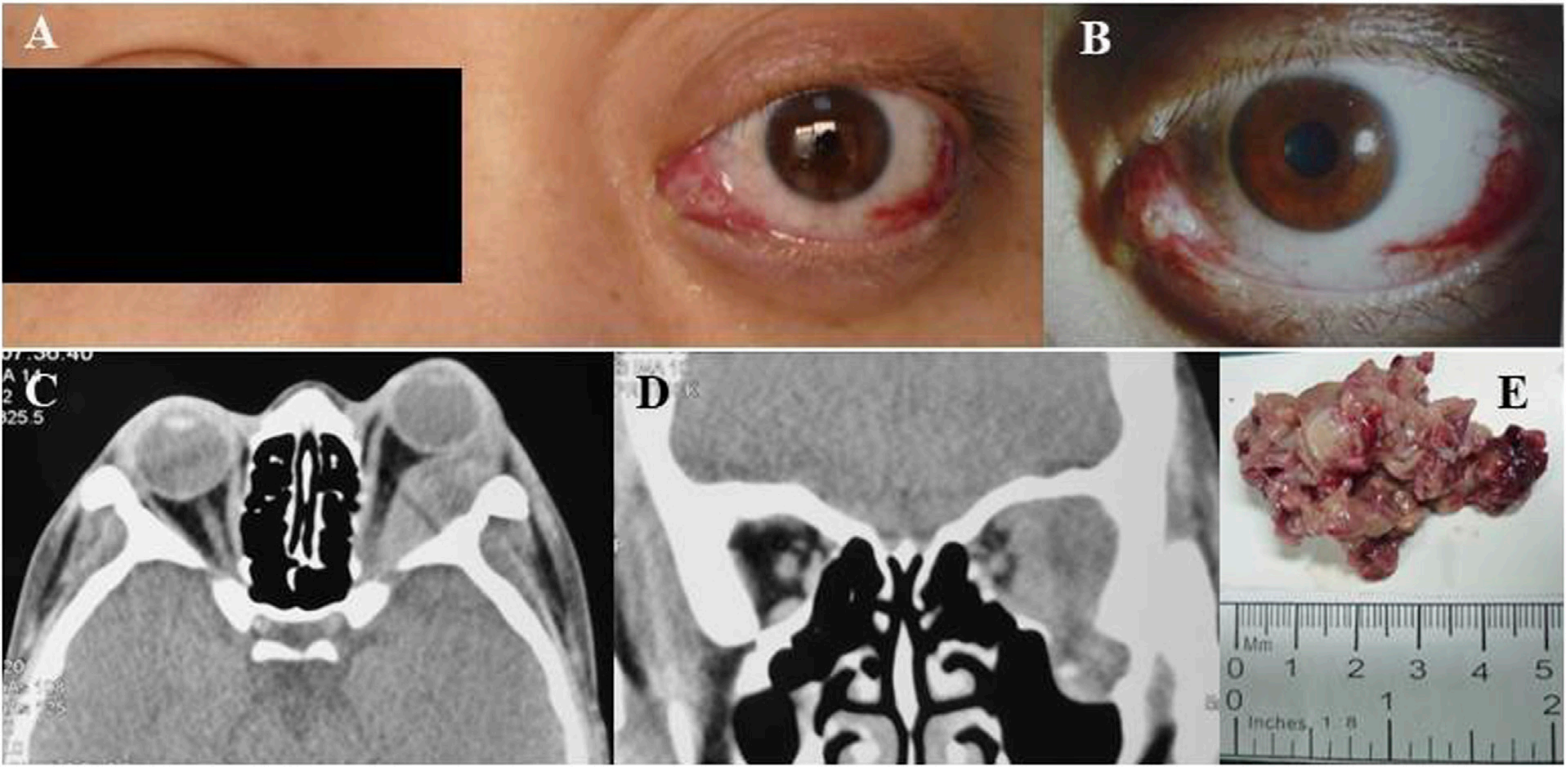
(A) Left preoptic bulge. (B) Left eye showing conjunctival congestion, edema and exophthalmos. (C) Axial CT scan of the orbits showing irregular mass in the left orbit with compression of the left orbital bulb, external rectus muscle and optic nerve nudged. (D) Coronal CT image revealed bone destruction was seen below the left eye and a partial mass invaded the temporal fossa. (E) orbital mass resected from patient 1.
